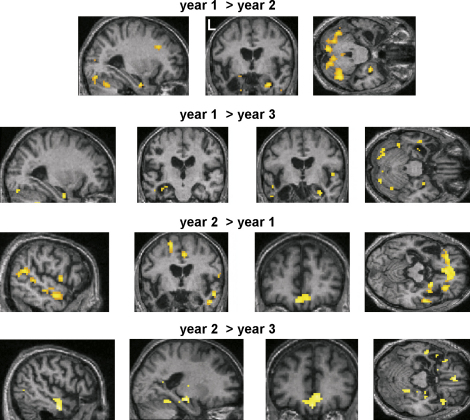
Direct comparisons across years for autobiographical memory. Functional images are shown on a selection of relevant sagittal, coronal and axial sections from the mean structural MRI scan of the patient averaged across the three years. See Table 5 for full details of exact contrasts and activation locations. Activations are shown at a threshold of *p* < 0.005 uncorrected. L: left side of the brain.
